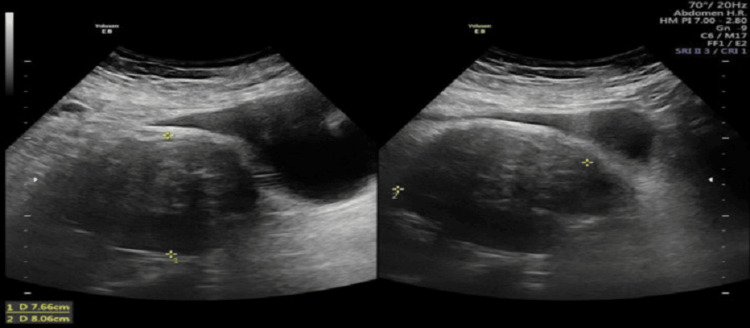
Ultrasound 25 days post-delivery involuted the uterus and decreased the size of the fibroid to 7.6 cm
